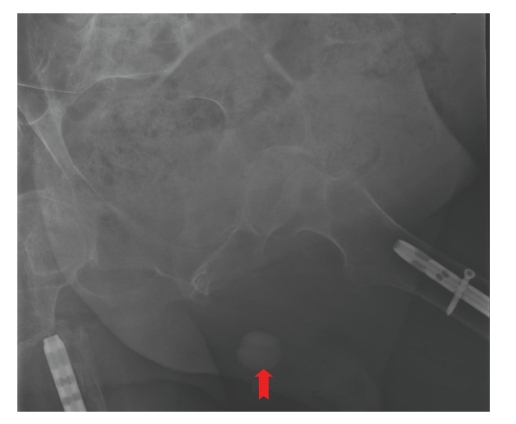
X-ray of pelvis was taken after injecting two mL of contrast through balloon channel of Foley catheter. The Foley balloon was located in scrotum (arrow) and not inside urinary bladder.
